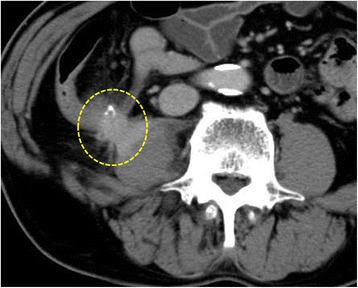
A computed tomography scan reveals a retroperitoneal abscess close to the ileocolostomy. The retroperitoneal abscess and fistula extends from the psoas abscess to the femoral abscess (*encircled by the dotted line*)
